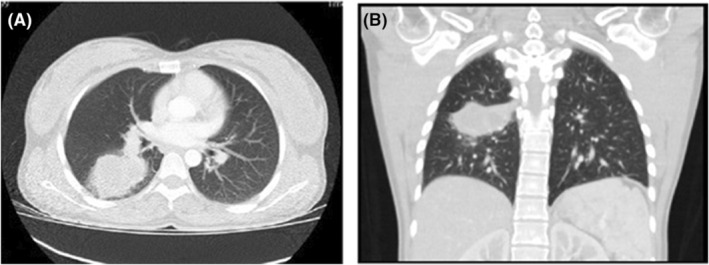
(A) Axial computed tomography view, shows an increase in the abscessed lesion in the upper segment of the lower lobe on the right side. (B) Coronal computed tomography view.
